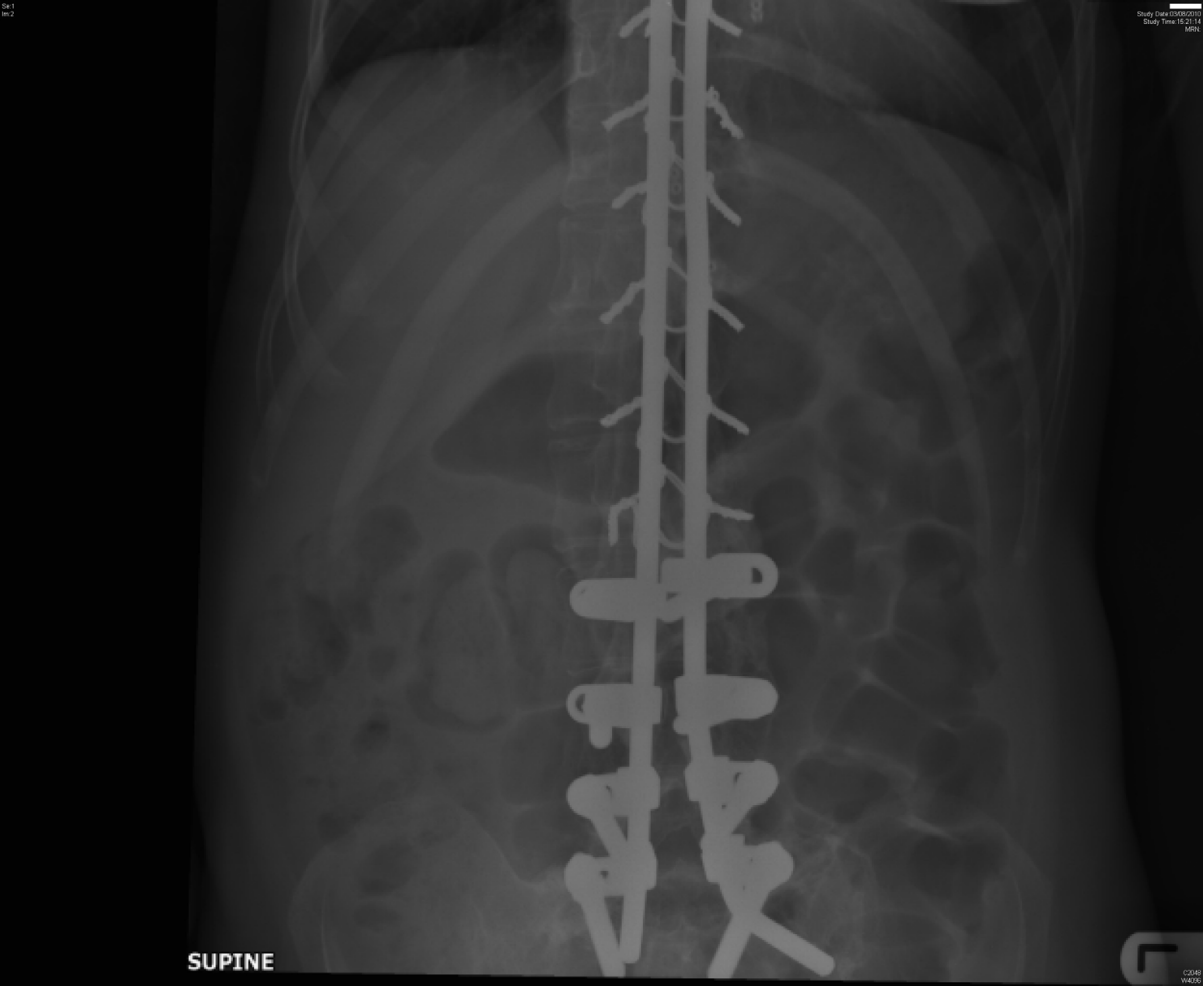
Case number: 3. X-ray of abdomen, taken on 03 August 2010, revealed fixation with spinal rods and pedicular screws from the level of T-2 to S-2 inclusive. Spinal fixation precluded administration of subarachnoid block.
